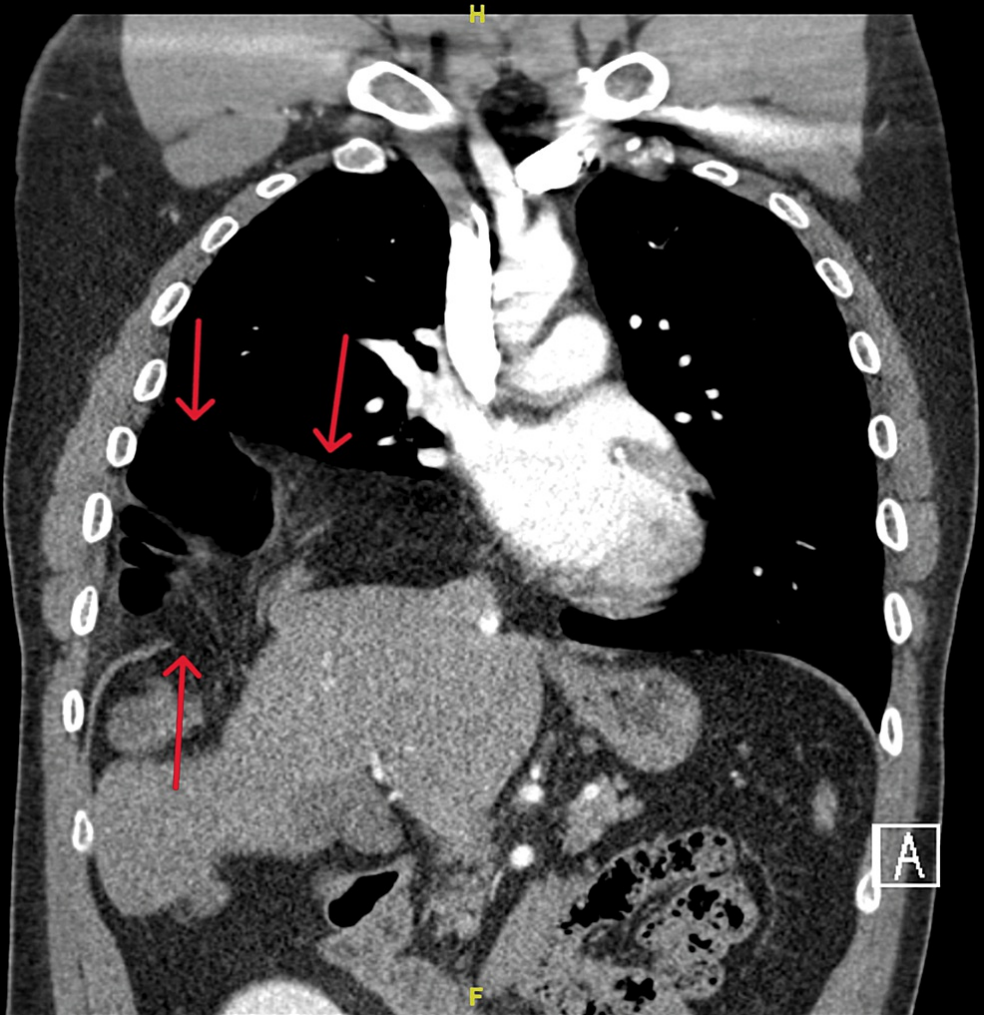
Coronal chest/abdomen CT showing right diaphragmatic hernia with incarcerated large bowel and liver and Chilaiditi sign. Red Arrows: Large Bowel
